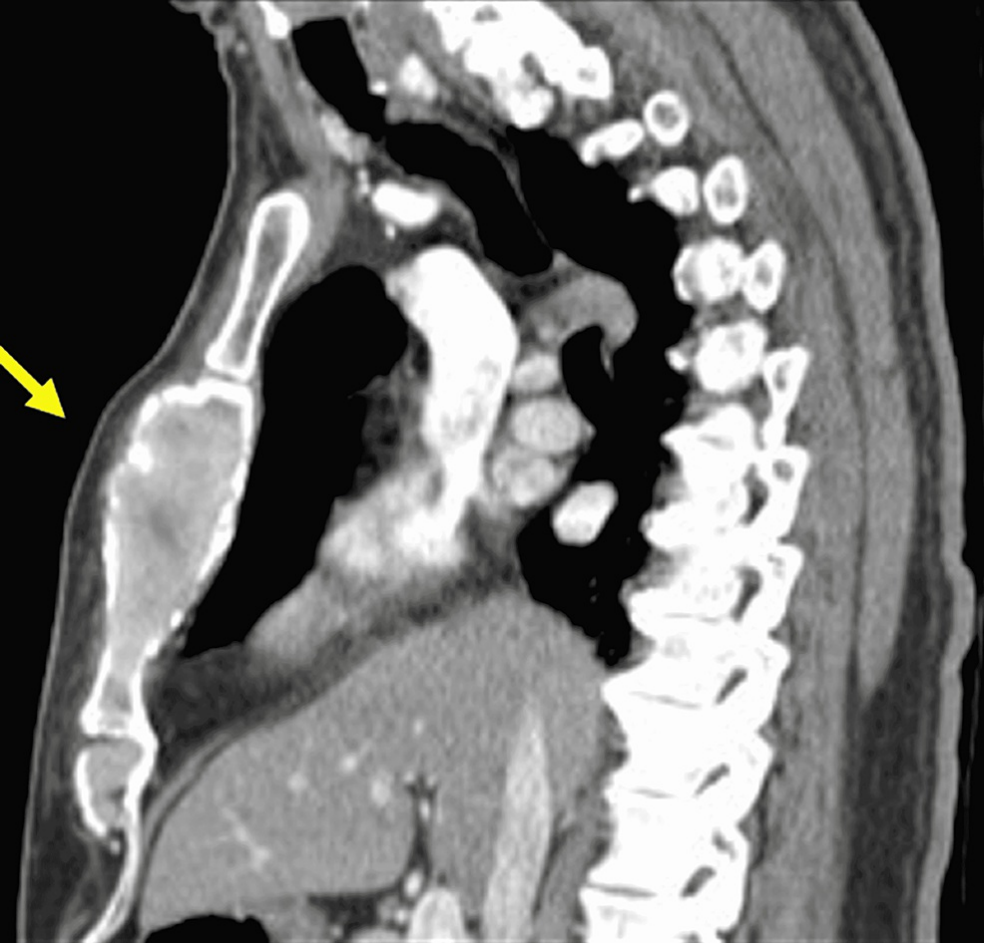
Sagittal computed tomography view of the sternal mass
